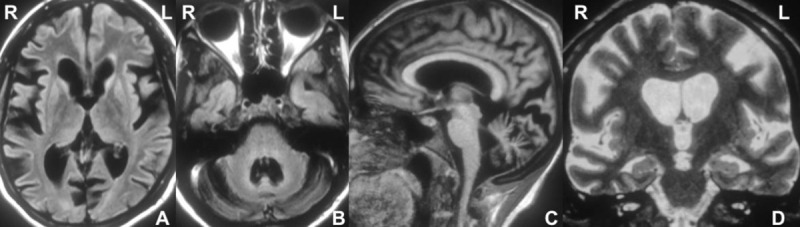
Brain MRI showing generalized atrophy and striatal hyperintensities (A), cerebellar atrophy and a widened fourth ventricle (B) and thinning of corpus callosum (C). Hippocampal atrophy and structural changes commonly seen in tauopathies are also seen (D).
